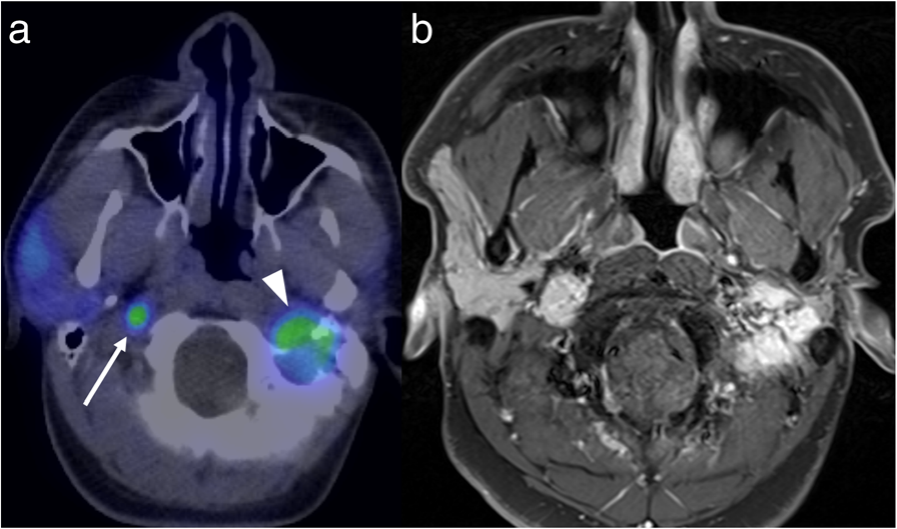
FDG-PET (a) performed for follow-up of a patient with a germline succinate dehydrogenase subunit B mutation and a known left glomus jugulare paraganglioma (arrowhead) demonstrates a new area of FDG-avidity just below the skull base on the right (arrow). The subsequent post-contrast MRI (b) supports that this is a new paraganglioma rather than a metastasis
